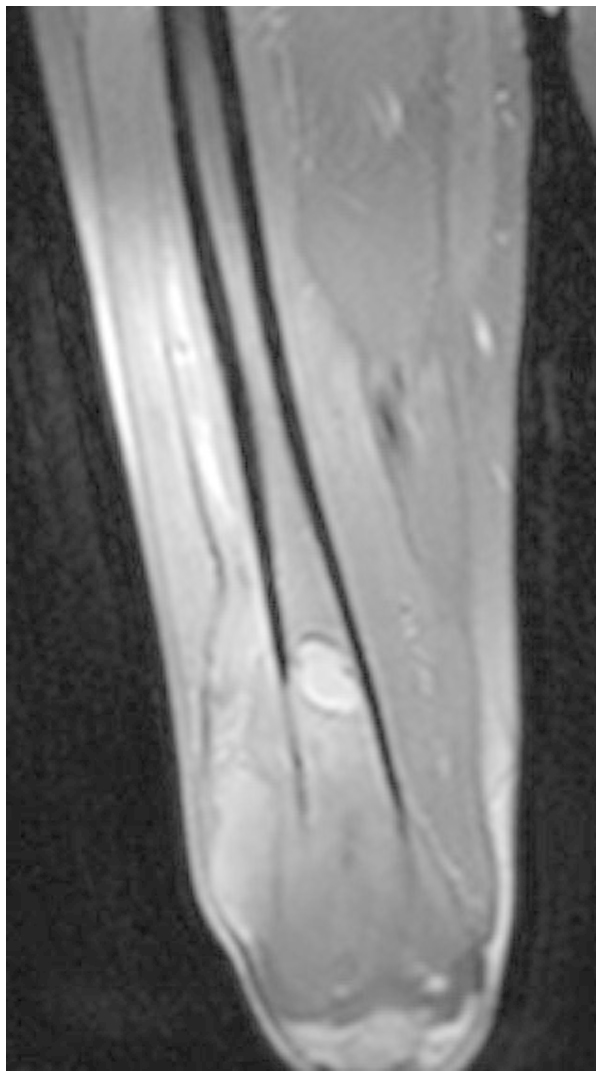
Magnetic resonance image demonstrating the osteolytic lesion in the femur and diffuse erosion of the cortex, with involvement of the surrounding soft-tissues.
